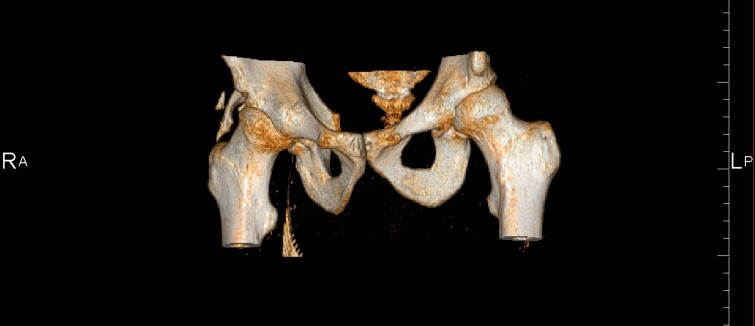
3D CT reconstruction of a 34-year-old triathlete showing grade 3 HO with acetabular origin and posterolateral location of the HO.
